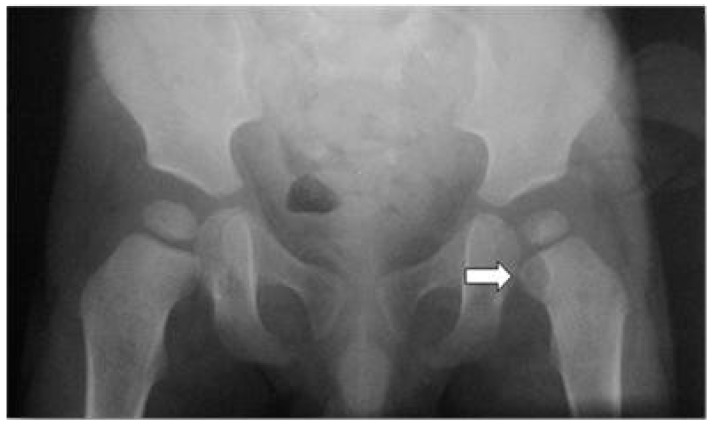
X-rays of the pelvis. Osteolytic lesion of the proximal metaphysis of the left femur (arrow).
